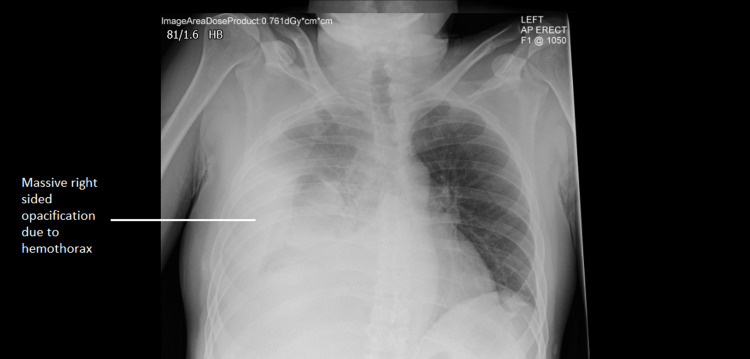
Chest X-ray showing massive right-sided opacification due to hemothorax
